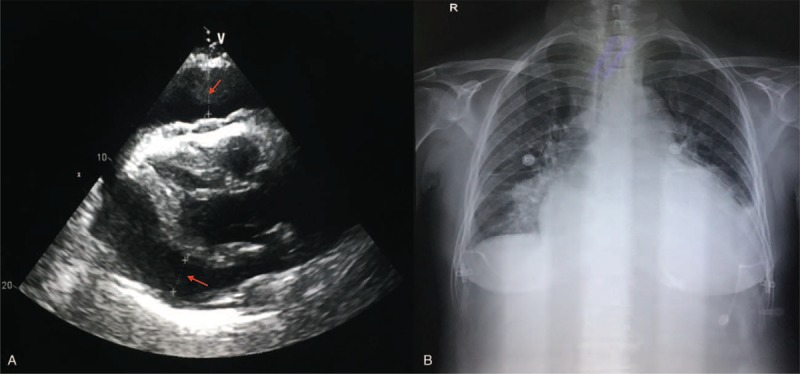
(A) Echocardiogram shows a large amount of pericardial effusion. (B) The chest X-ray reveals prominent cardiomegaly, with evidence of pericardial effusion.
